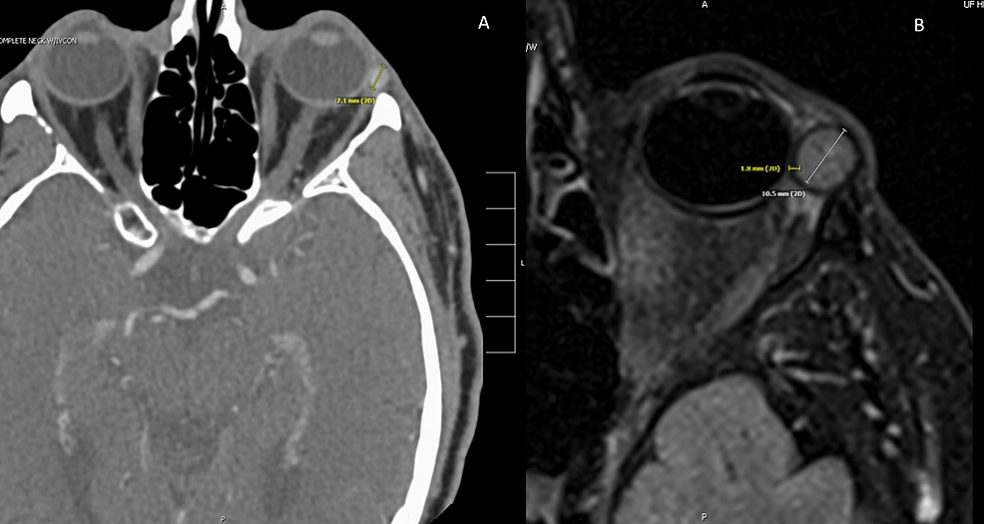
CT image (A) and MRI image (B) of left orbital mass
